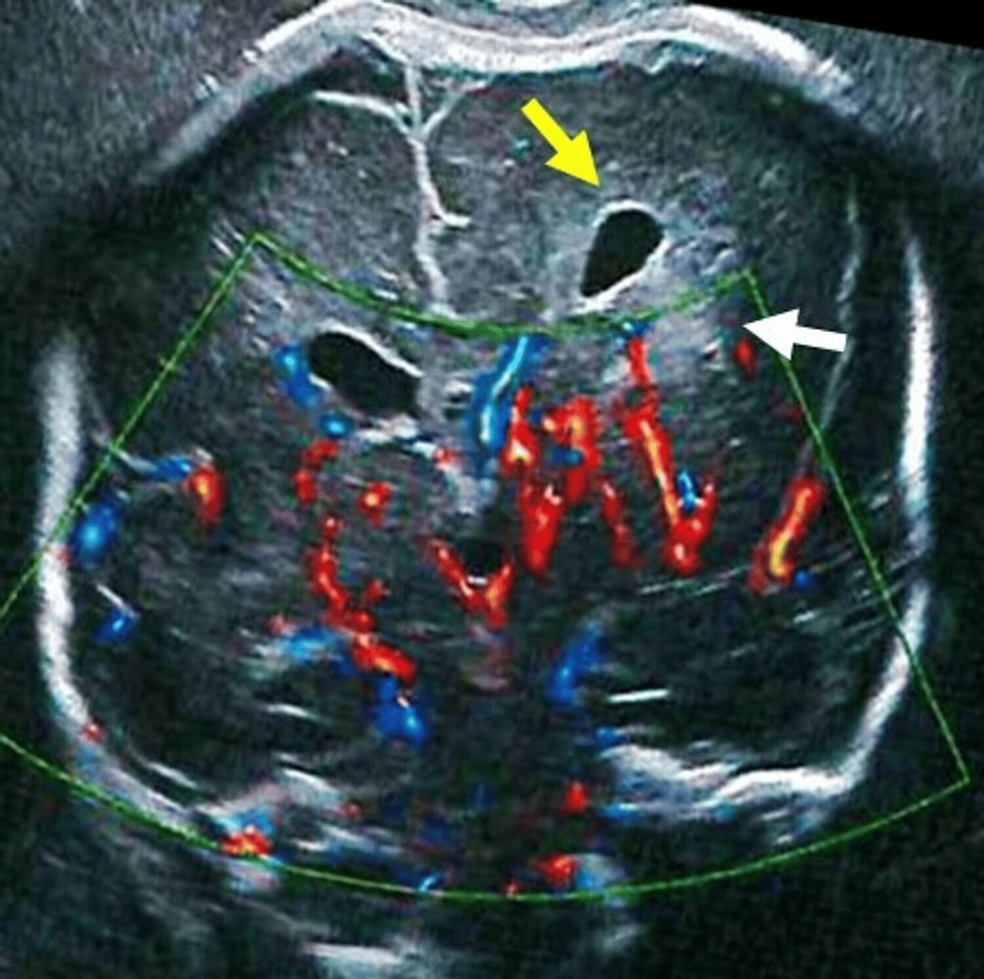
Prenatal ultrasound, coronal section of the brain. Dilatation of the left-sided lateral ventricles (yellow arrow), with hyper-echogenicity of the adjacent white matter (white arrow).
